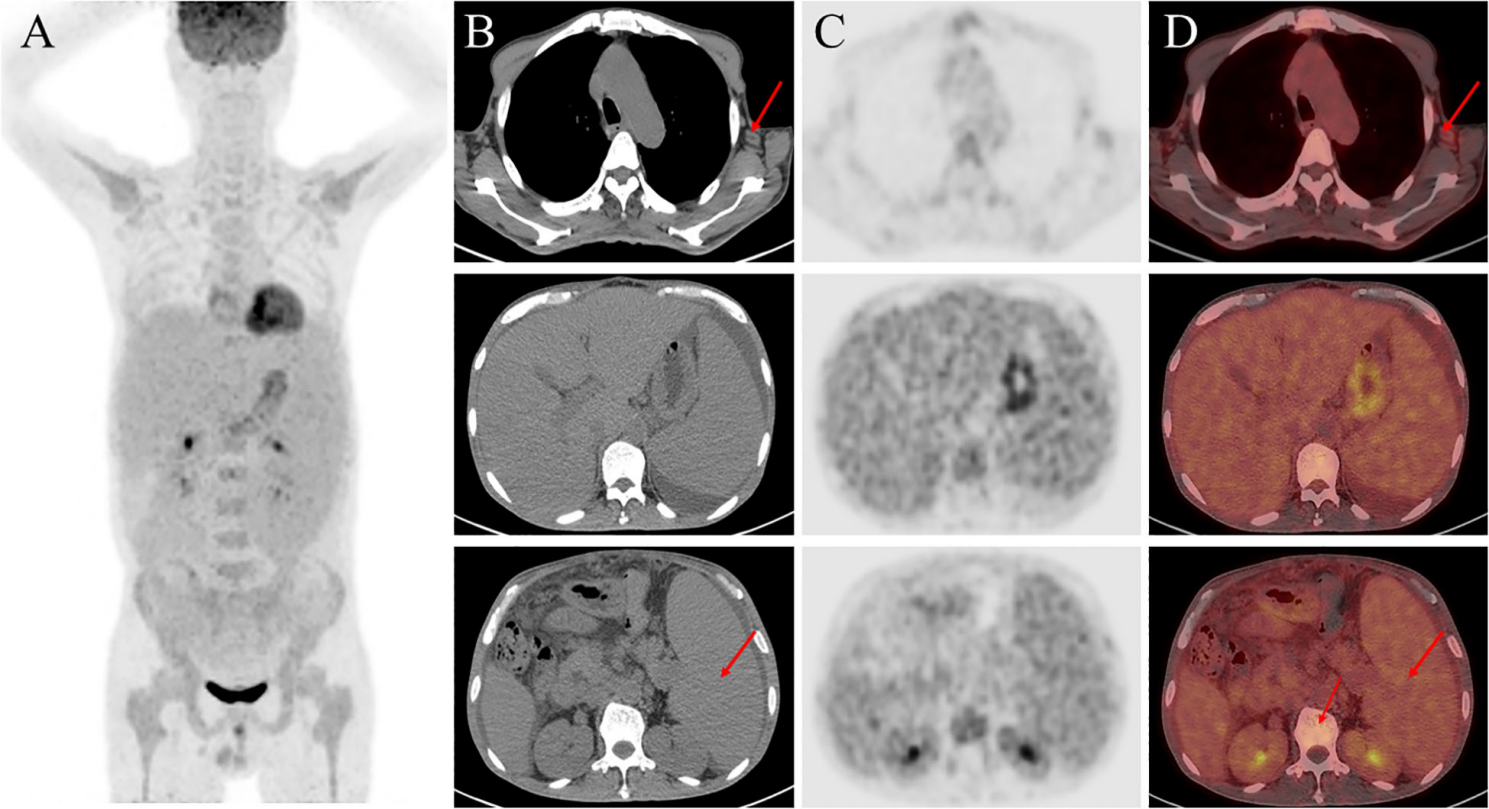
^18^F-FDG-PET/CT imaging. (A) Maximum intensity projection (MIP) images of the chest, upper abdomen, and lower abdomen, respectively (left to the right); (B) PET images; (C) Fused PET/CT images; (D) CT images.
